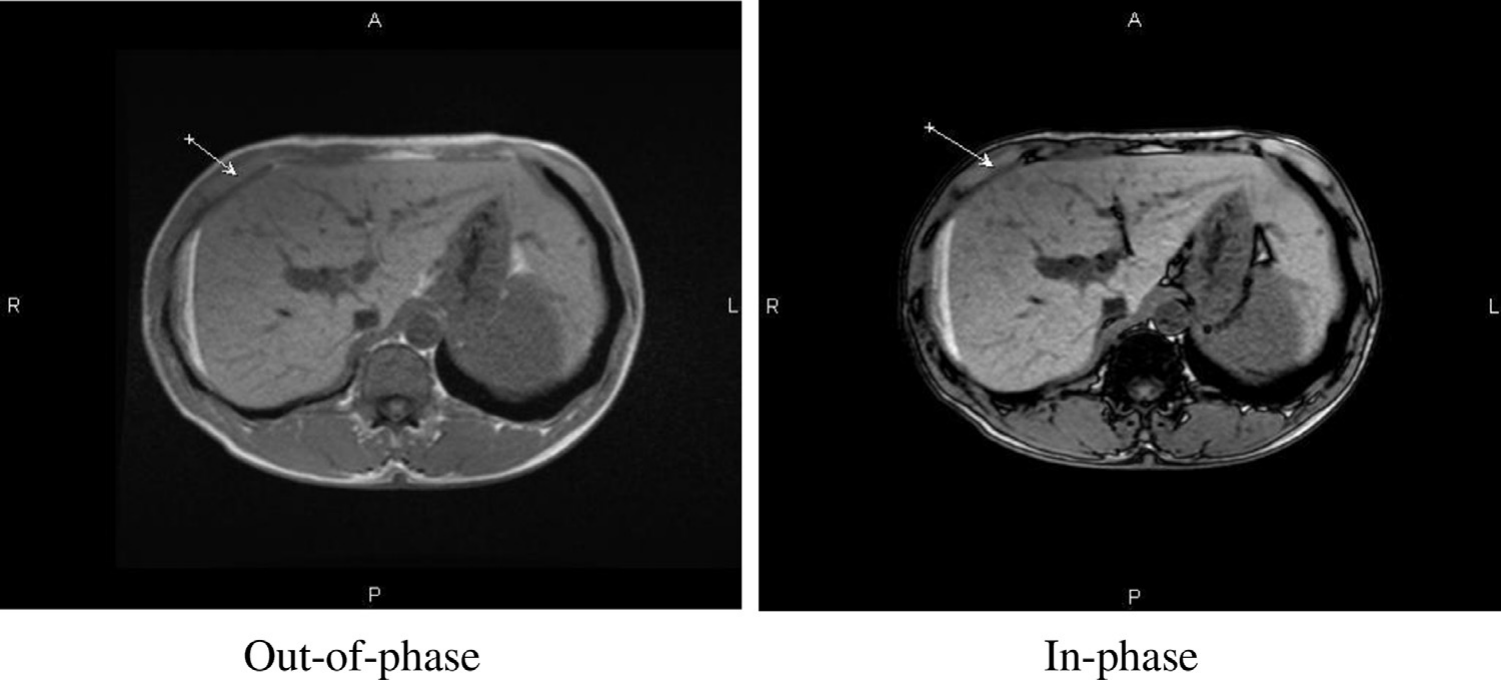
MRI shows hypointense hepatic foci (arrow) with decreased signal intensity on the out-of-phase image (B) compared to the in-phase image (A), indicating intralesional fatty substance.
